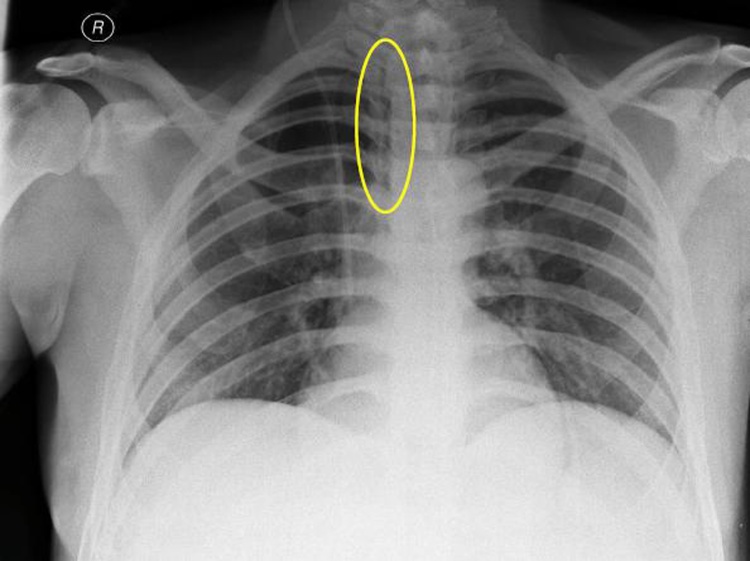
Chest X-Ray. Small amount of air in the mediastinum next to the upper third of the trachea indicating pneumomediastinum.
